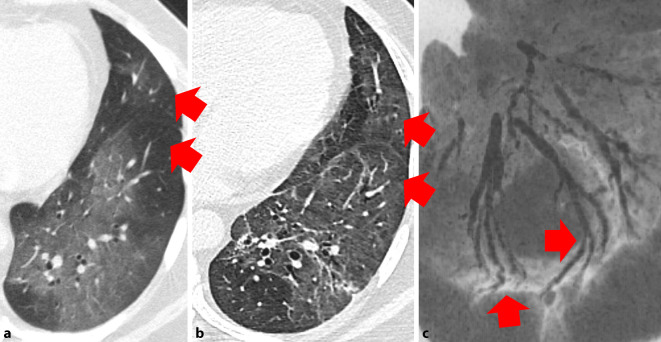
### CT-Veränderungen der Lunge bei Post-COVID-19-Syndrom
